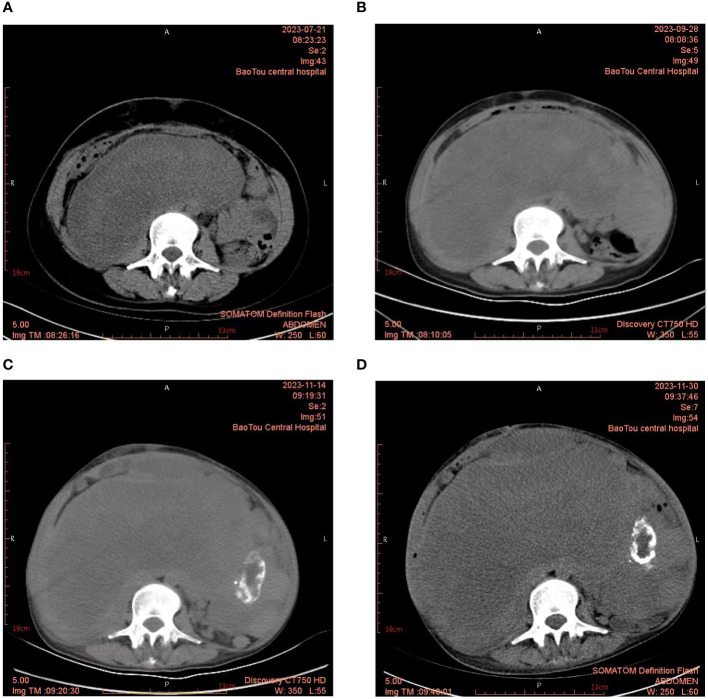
(A–D) Abdominal CT showed multiple huge masses in the abdominal cavity. By the eighth surgery, the larger masses had grown to 23×12 cm.
